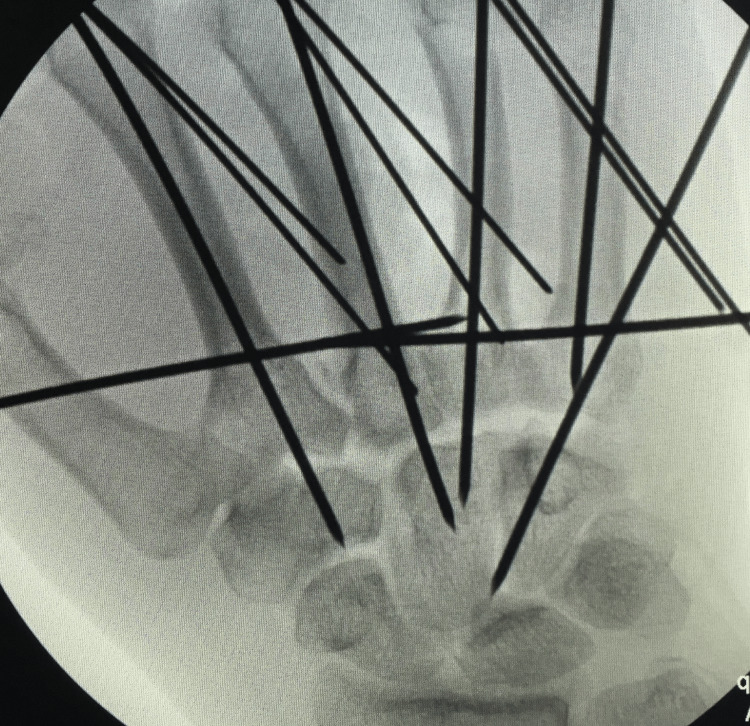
Postoperative radiographs of the right wrist Anteroposterior radiograph of the right wrist demonstrating fixation of the CMC dislocations and metacarpal fractures with multiple Kirschner wires. CMC, carpometacarpal
